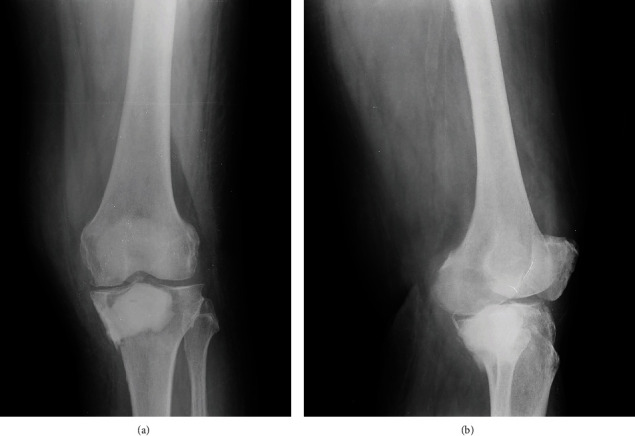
Plain radiograph ((a) anteroposterior, (b) lateral) at 12 months postoperatively showing containment of the PMMA bone cement and intact proximal tibia.
